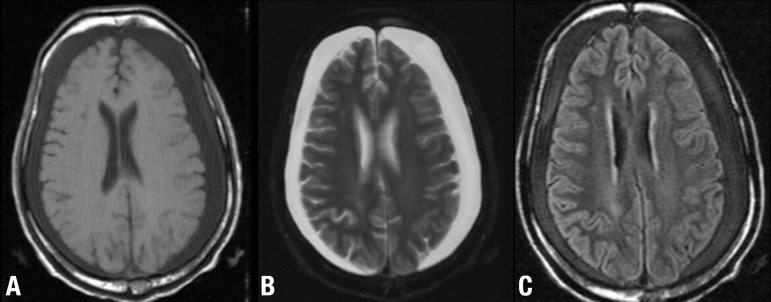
Cognitive decline 8 weeks after TBI. Axial T1-weighted [A], T2-weighted
